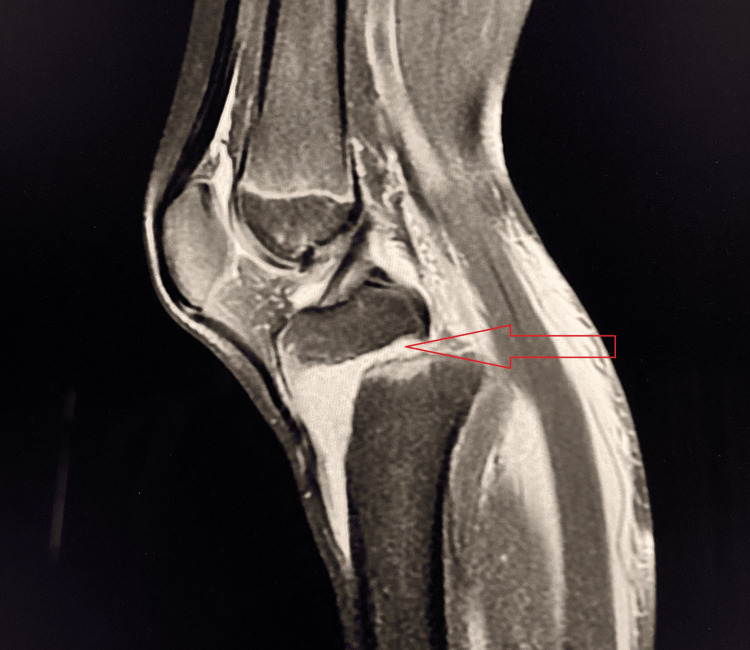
Salter-Harris Type III physeal fracture Sagittal PD-FS MRI demonstrates a fracture line (red arrow) extending through the epiphysis and growth plate, with anterior displacement of the distal fragment and moderate joint effusion. PD-FS, proton density fat-suppressed
